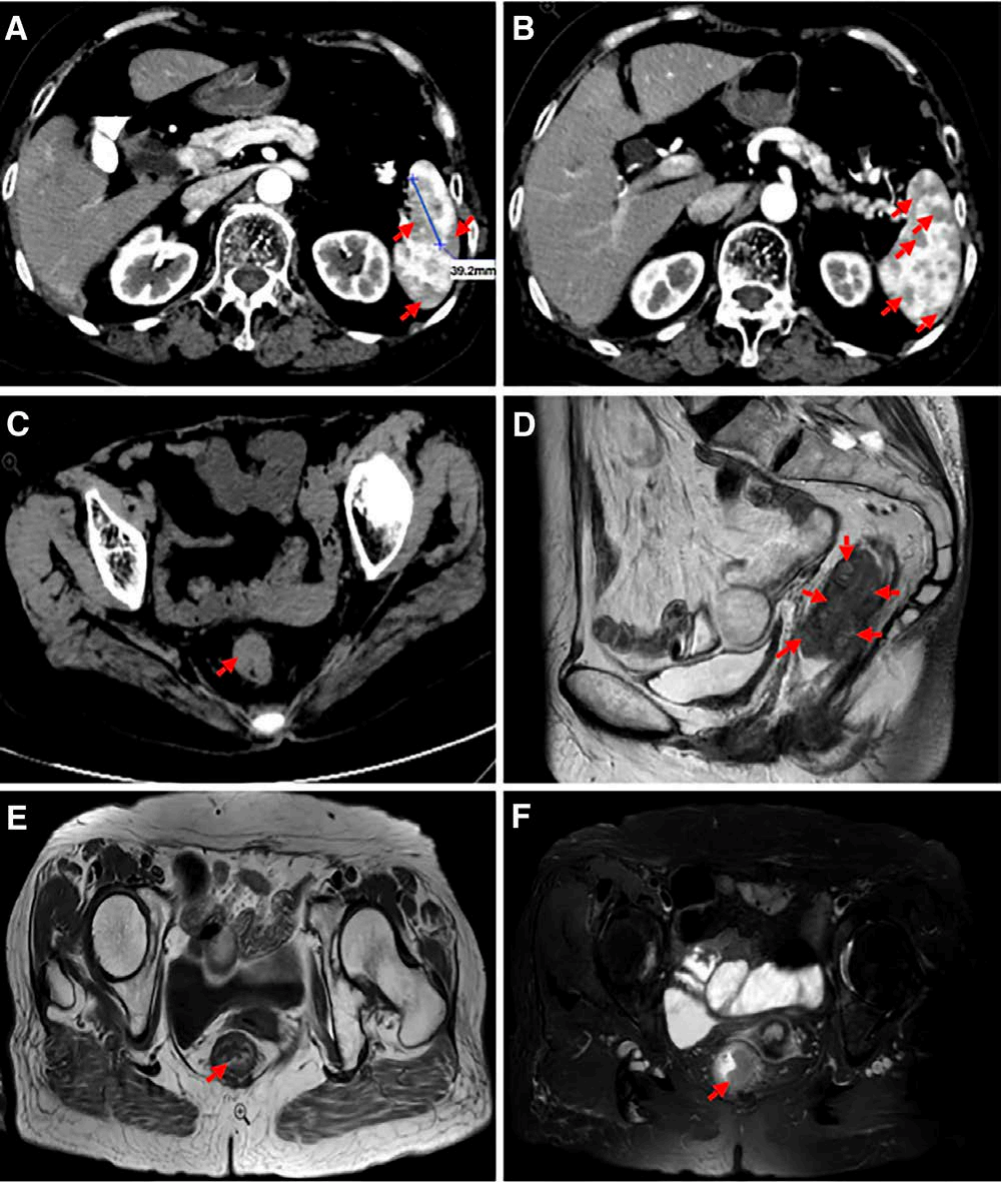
Imaging examinations of the spleen and the rectum. (A and B) Abdomen enhanced CT showing multiple low-density shadows in the spleen (red arrow), the largest diameter of which is 39.2 mm (blue lines). (C) Abdomen CT showing wall thickening of the upper rectum (red arrow). (D) MRI (T2W-TSE-HR) showing that the cumulative length of the tumor was about 57 mm, the lower margin of the tumor was higher than the rectal ring, and the distance from the anorectal ring was about 20 mm (red arrow). (E) MRI (TSE-axial) showing irregular wall thickening of the middle and upper rectum, and the rectal lesion is about 59 mm from the anal margin (red arrow). (F) MRI (T2W-SPAIR-tra) showing a slightly high signal of the rectal lesion (red arrow). CT = computed tomography, MRI = magnetic resonance imaging.
